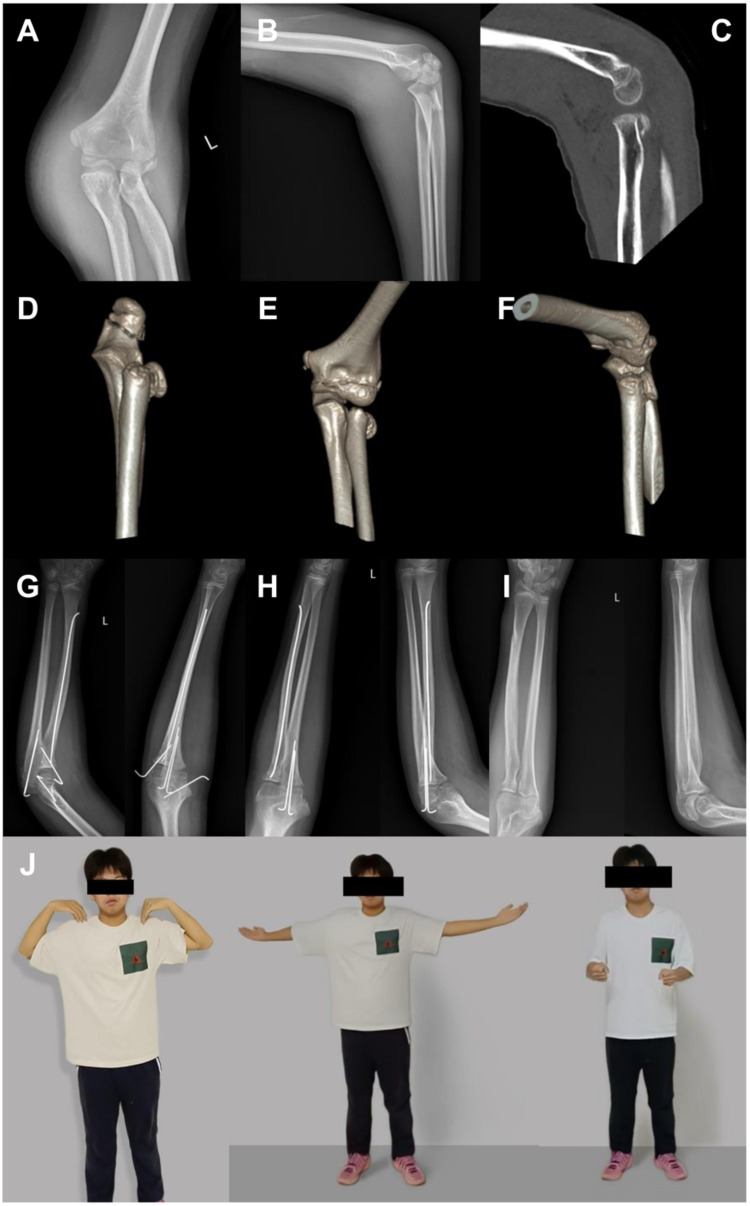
Representative case of an unstable pediatric radial neck fracture treated with elastic intramedullary nailing combined with Kirschner wire fixation. (A,B) Preoperative anteroposterior and lateral radiographs showing a severely displaced radial neck fracture. (C–F) Preoperative computed tomography images demonstrating marked fracture displacement and collapse of the radial neck, associated with olecranon and medial epicondyle fractures. (G) Radiograph at 1 month postoperatively showing maintained reduction and early callus formation. (H) Radiograph at 2 months postoperatively demonstrating progressive fracture healing. (I) Radiograph at 8 months postoperatively confirming complete fracture union. (J) Clinical photograph at final follow-up showing full elbow flexion, extension, and forearm rotation.
